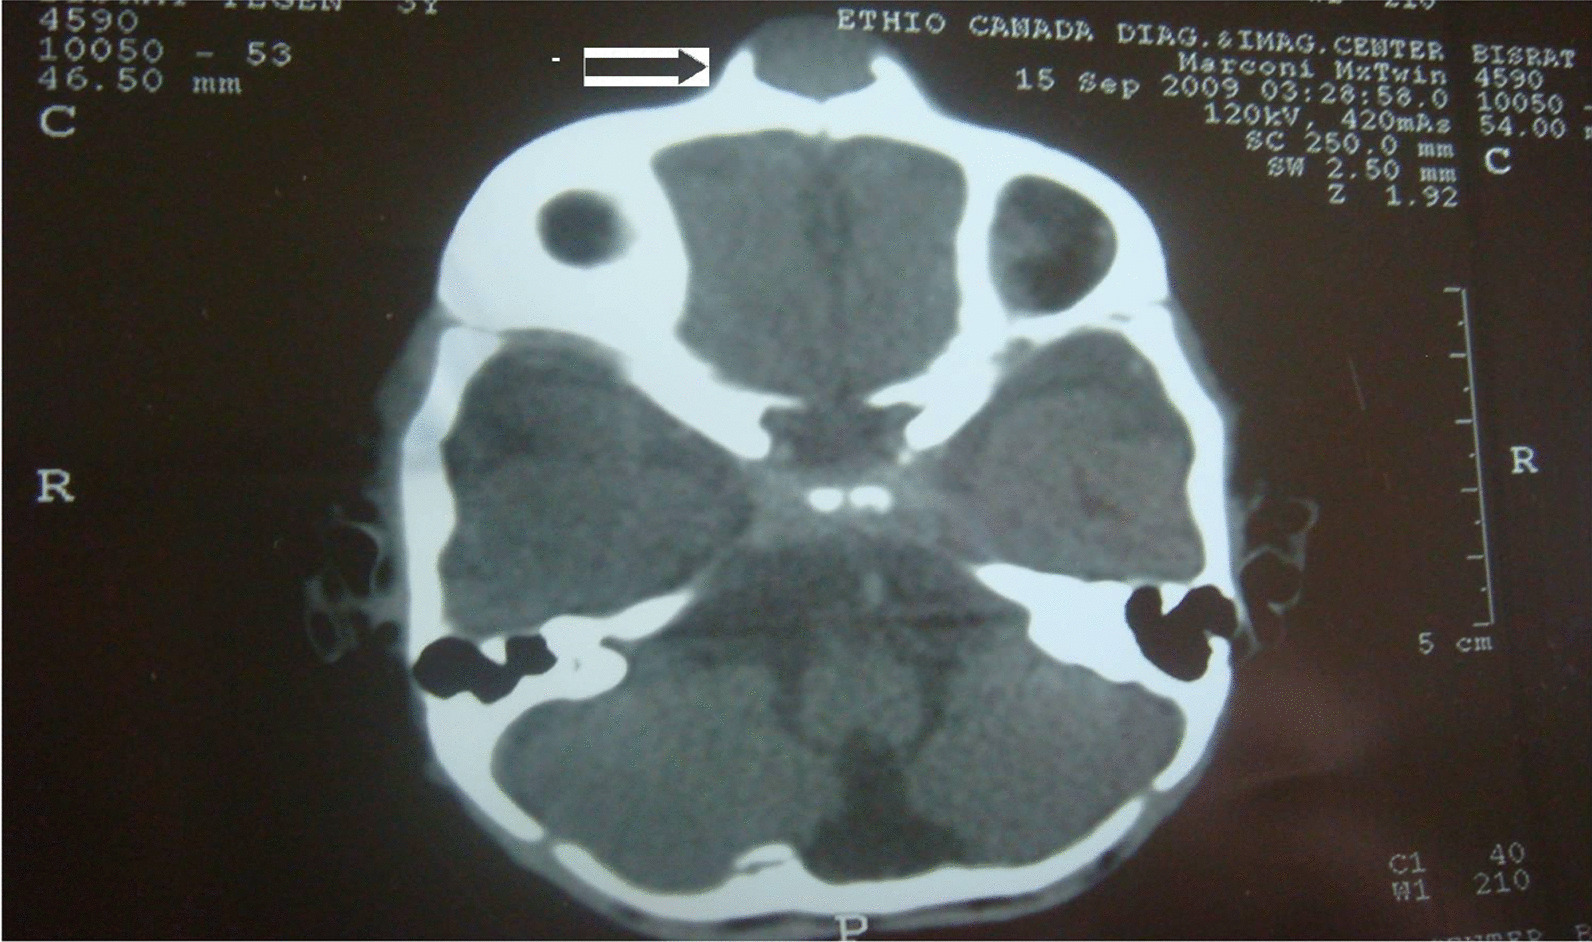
Computed tomography showing forehead mass with peripheral bone cuffing (hyperostostic) (arrow)
